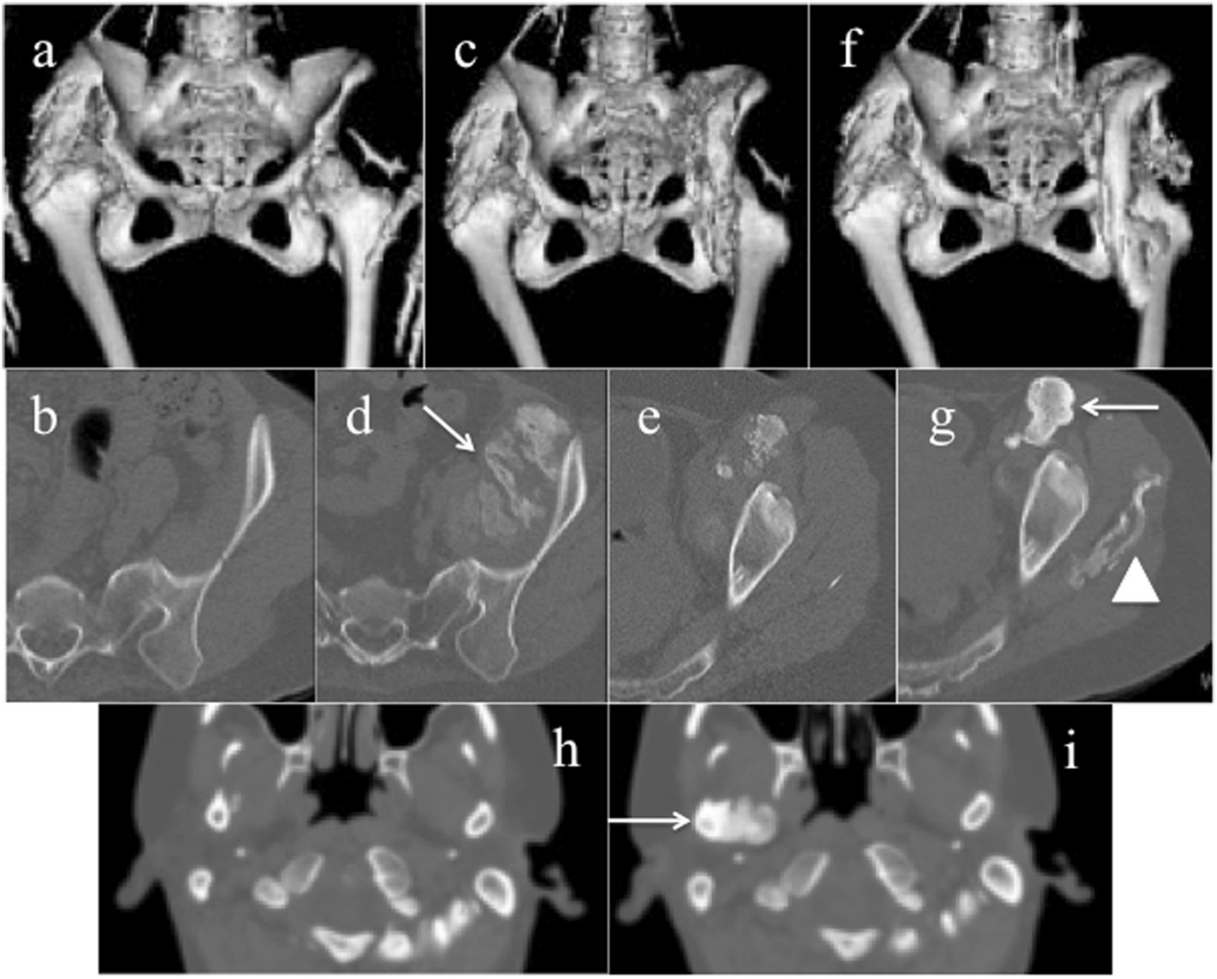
CT images form Case 3. Reconstructed 3D-CT images of the hip joints at baseline (a), at M-12 m (c), and at D-12 m (f). Axial CT images of the left pelvis at baseline (b), at M-12 m (d, e), and at D-12 m (g), and those of the bilateral jaws at M-12 m (h), and at D-12 m (i). Massive new bone formations in the left iliac muscle were developed during the medication phase (d, arrow). During the discontinuation phase, the left intra-iliopsoas ossification matured (g, arrow), and heterotopic boned in the left gluteus medius (g, arrow head) and the right jaw joint (i, arrow) were newly formed.
